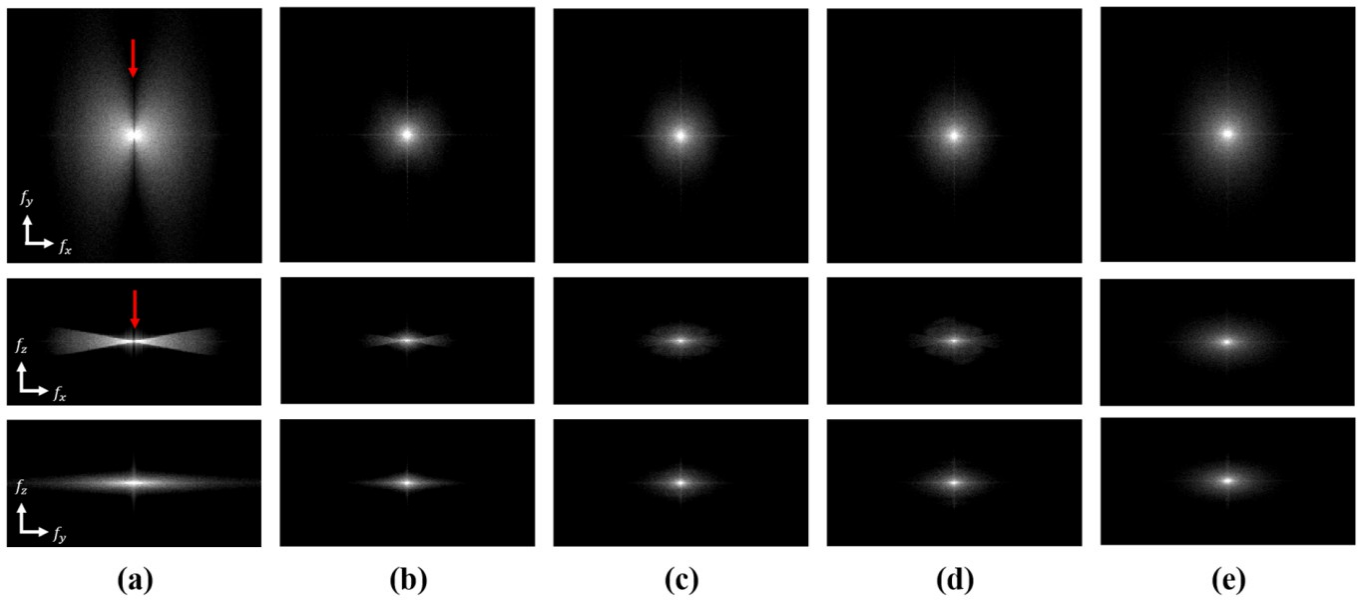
Frequency domain analysis. Frequency responses of DBT images using FDK, deblurred images, and CBCT images for *f*_*x*_-*f*_*y*_ plane (Top), *f*_*x*_-*f*_*z*_ plane (middle), and *f*_*y*_-*f*_*z*_ plane (bottom). (a) DBT images with FDK reconstruction, (b) deblurred images after Phase 1 and deblurred images after Phase 2 with (c) MAE, (d) PL-MAE, and (e) CBCT images. The display window is [1 4]. The red arrows indicate the missing data regions in the DBT images.
